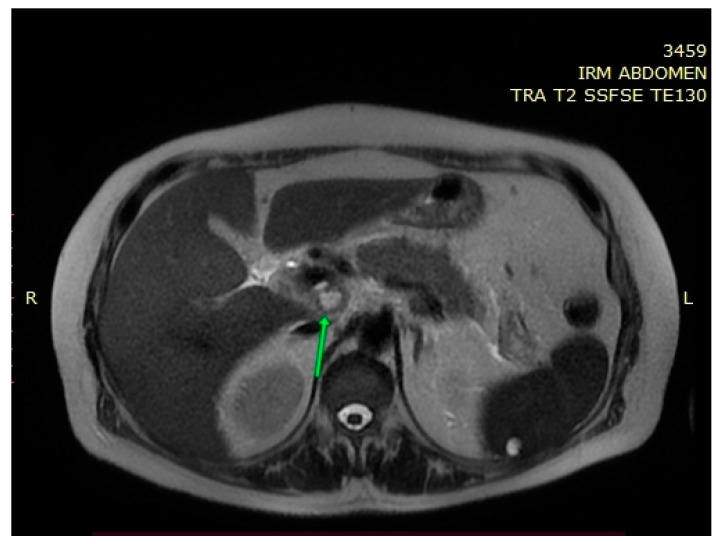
MRI—T2 weighted image: a multicystic cephalopancreatic lesion.
